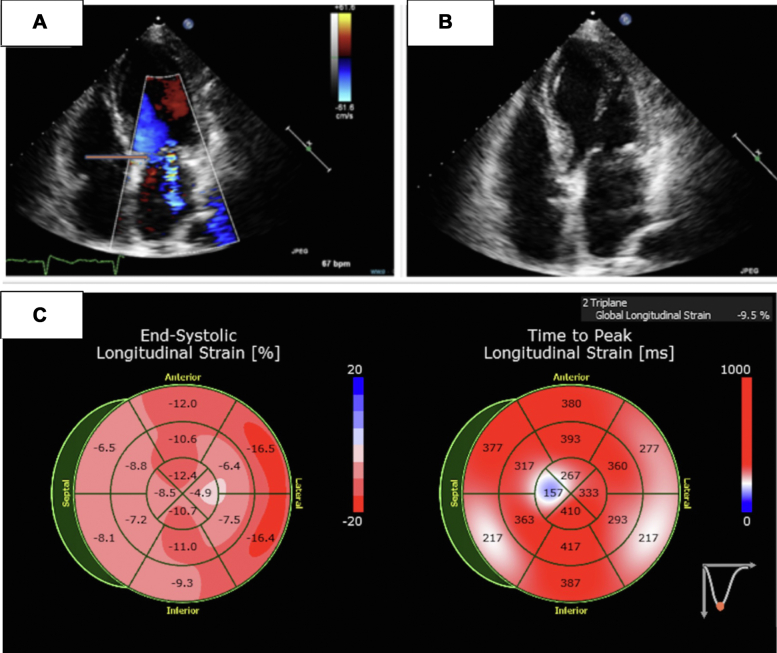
Transthoracic Echocardiogram on Osimertinib Transthoracic echocardiography reveals a dilated left ventricle (A, B) accompanied by moderate secondary mitral regurgitation, as indicated by the arrow in (A). Additionally, echocardiography (C) shows a reduced global longitudinal strain of −9.5%.
